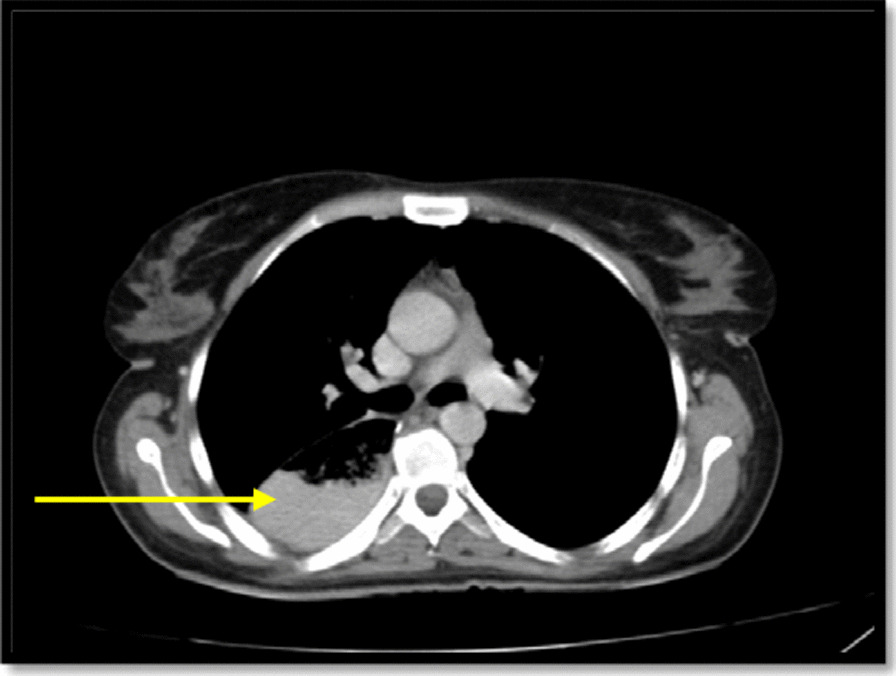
Spiral chest CT scan: pulled-up stomach at the right paraspinal region shown with a yellow arrow
